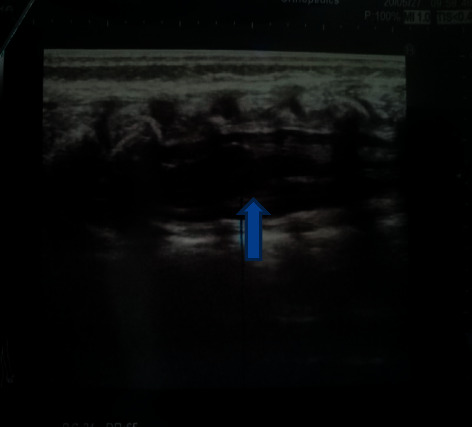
Ultrasound view: abrupt termination of spinal cord at the L1 vertebra level (arrow) and thickened conus medullaris.
